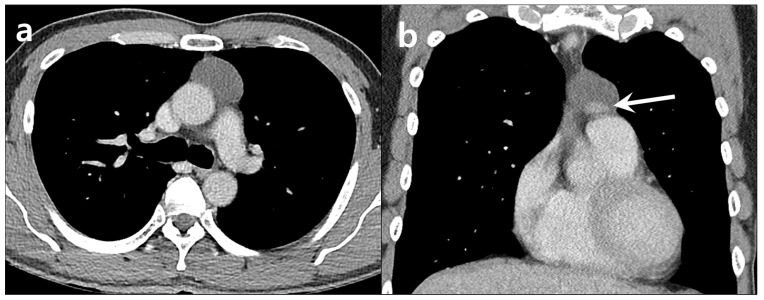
CT images of a 57-year-old man who was referred for surgical resection of an anterior mediastinal mass initially considered to represent a thymic cyst at an outside institution. (a) Axial contrast-enhanced CT image demonstrates an approximately 4 cm cystic lesion in the anterior mediastinum with a barely perceptible thin wall. (b) Coronal CT image reveals a small oval soft-tissue density (arrow) along the inferior aspect of the cystic lesion, raising concerns for a solid tumor component, an intracystic hemorrhage, or concentrated proteinaceous content [1,2].
